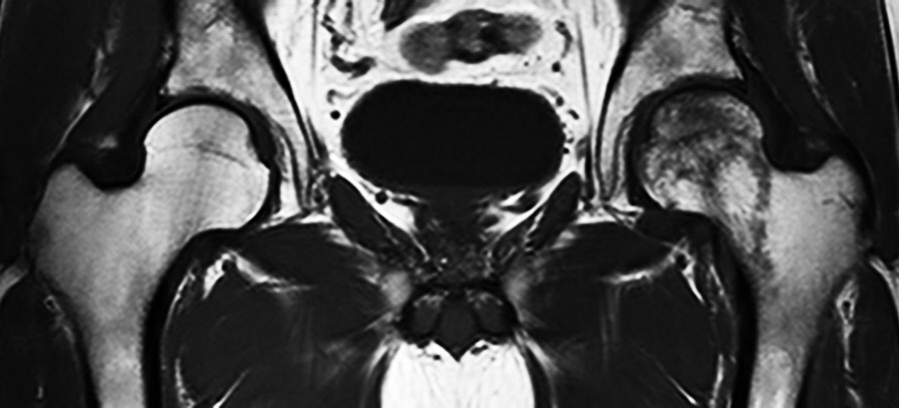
A coronal T1-weighted magnetic resonance image obtained 8.5 months after the initiation of corticosteroid therapy for purpura nephritis. Note the low-intensity band lesion in the left femoral head but no obvious abnormality in the right femoral head
